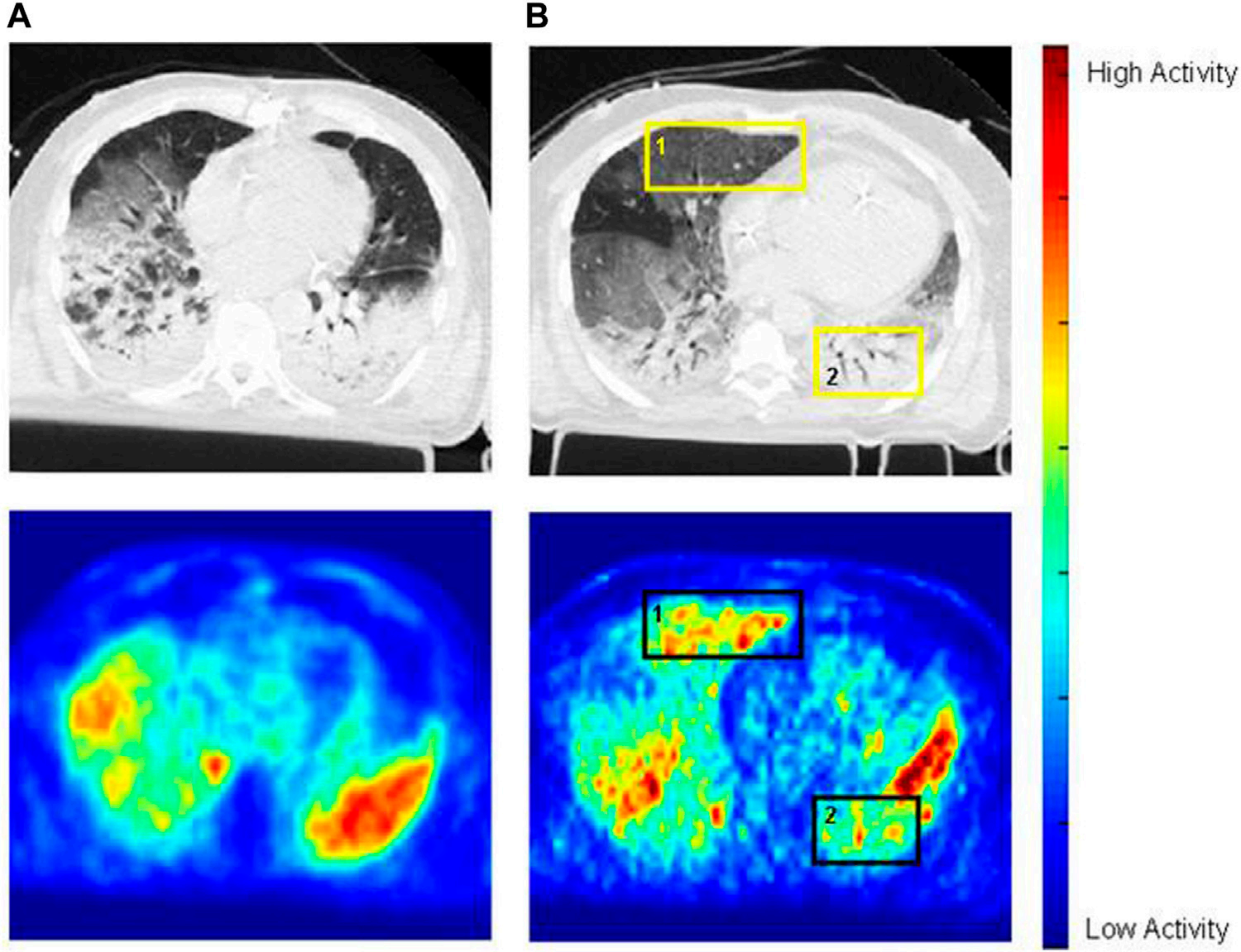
Images of cross-registered CT (top row) and [^18^F]FDG PET (bottom row) in two mechanically ventilated patients with ARDS. In patient A (images on the left), [^18^F]FDG distribution parallels that of the opacities on CT. In patient B (images on the right), [^18^F]FDG uptake is higher in normally aerated regions than in dorsal opacified regions (e.g., square 1 *versus* square 2, or ventral half of the left lung *versus* its dorsal half–square 2). Reprinted from Bellani et al. (2009), with permission.
